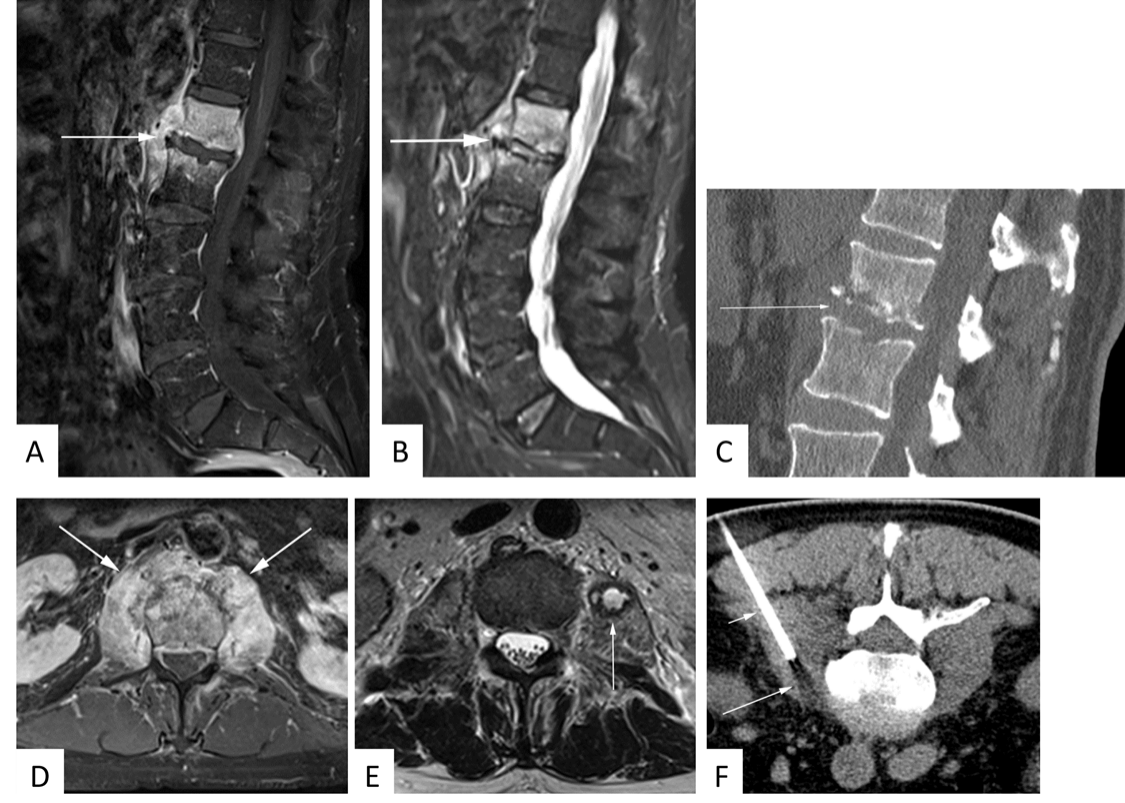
63 year-old male patient with suspected spondylodiscitis. Sagittal T1-weighted fat-saturated contrast-enhanced sequence (A) demonstrates florid enhancement of adjacent vertebral bodies and paravertebral soft tissue. Note concomitant T2-hyperintensity of the disk space as well as vertebral and paravertebral edema on sagittal STIR image (B). Sagittal CT reconstructions reveal arrosion of adjoining end-plates (C). Paravertebral soft tissue enhancement is even more conspicuous on contrast-enhanced axial T1-weighted fat-saturated sequence (D). An abscess is identified in the left psoas muscle as T2-hyperintensity surrounded by a T2-hypointense rim (E). CT-guided biopsy of the psoas abscess disclosed methicillin-resistant staphylococcus aureus infection (F).
